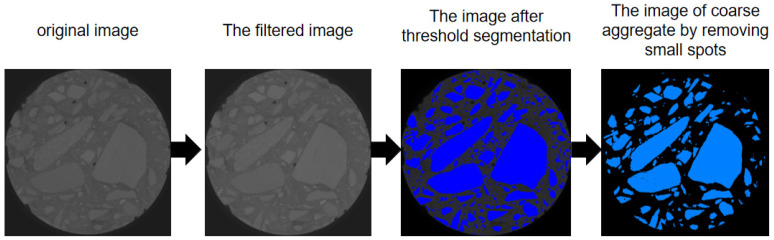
The processing steps of the CT images.
